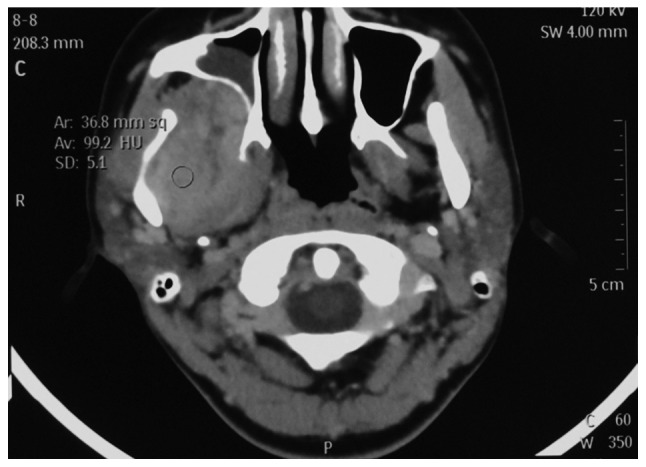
Axial computed tomography (CT) scan revealing a soft-tissue mass in the right infratemporal fossa (ITF), compressing the posterolateral wall of the right maxillary sinus and causing deformity.
